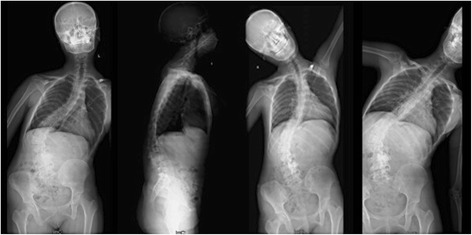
Posterior-anterior radiograph showed a 71 ° coronal plane deformity with an apex in the intervertebral space of L1 and L2. No significant reduction was found in side-bending image. The thoracic curve can be compensated with a Cobb’s angle of 43°. There is a 10.3 cm distant from the mid-point of superior border of sacrum to the C7 plumb line. This prompted severe coronal imbalance. Lateral radiograph showed sagittal imbalance (6.3 cm) and a 44° thoracolumbar kyphosis (T10-L2)
